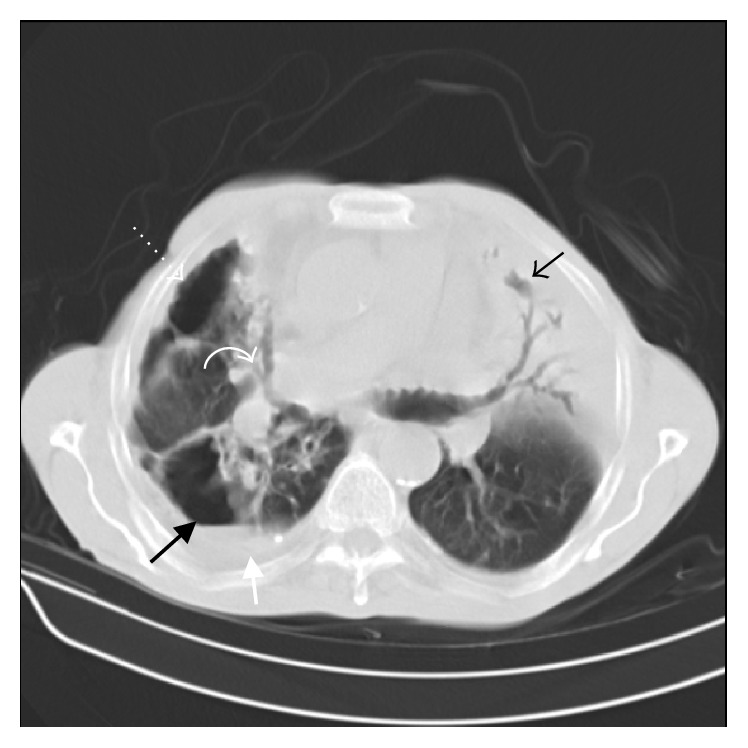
75-year-old male. Spiral CT scan at the level of pulmonary artery (lung window). Note consolidation with air bronchogram in lingula (black arrow) and air-fluid containing cavity in right lower lobe (thick black arrow) adjacent to pleural effusion (thick white arrow). There are also bronchiectasis in right middle lobe (curved arrow) and pneumothorax (dotted arrow).
